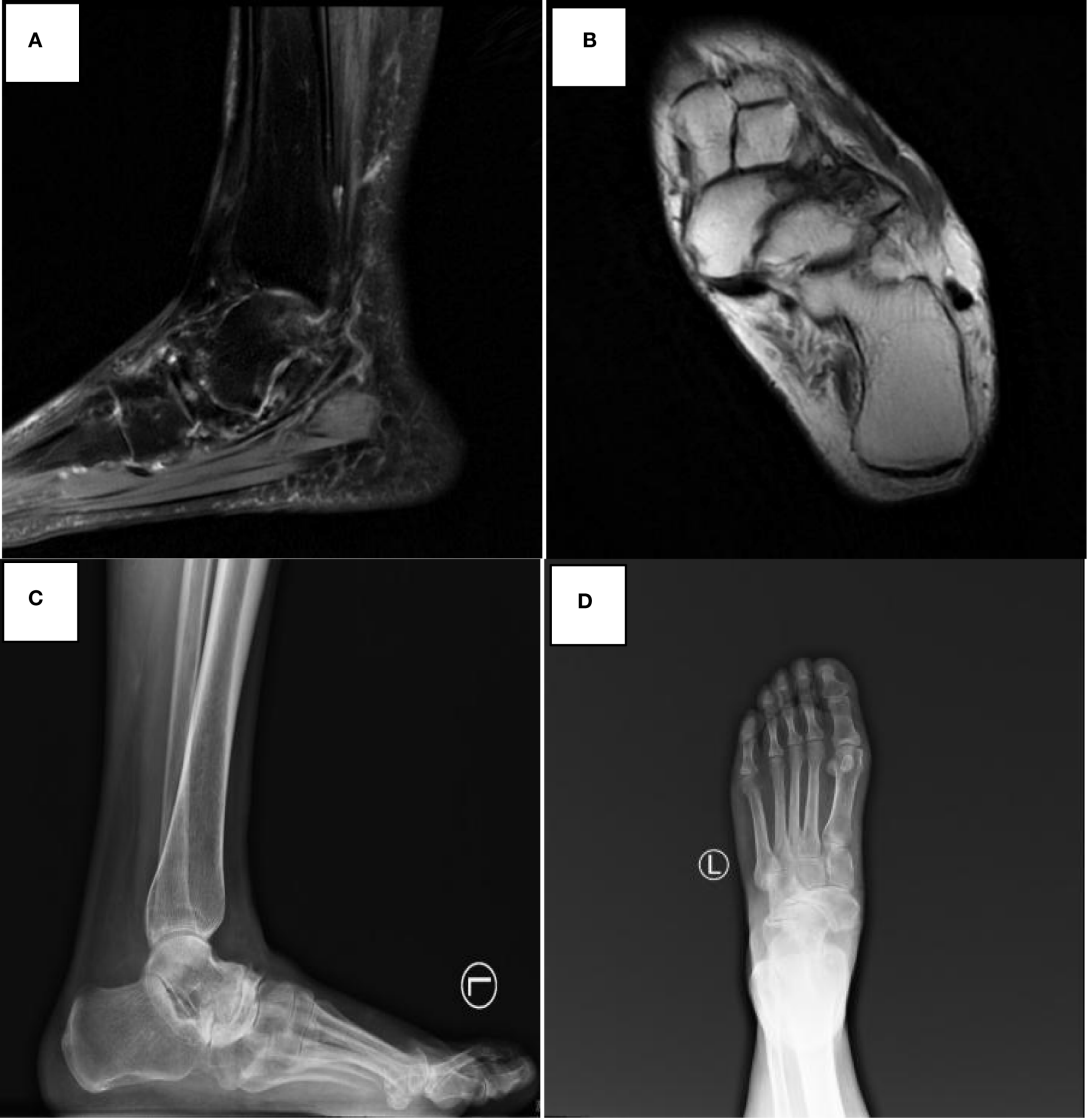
Preoperative imaging of navicular avascular necrosis in Muller-Weiss disease. (A, B) Preoperative magnetic resonance imaging demonstrates lateral compression and collapse of the navicular bone with avascular necrosis, subchondral cystic changes, and bone marrow edema, confirming Muller-Weiss disease. (C, D) Preoperative weight-bearing radiographs showing characteristic navicular deformity and talonavicular joint incongruity.
